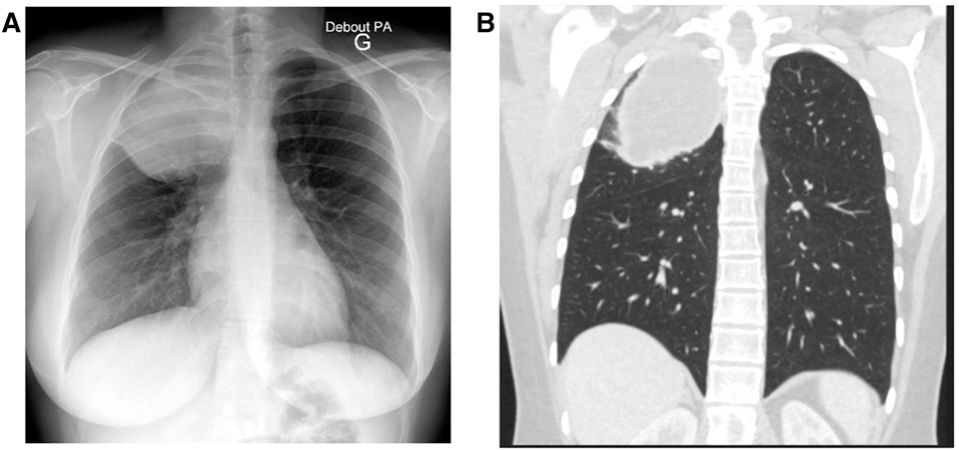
Chest radiography (Panel A) and computed tomography scan (Panel B) showing a cyst-like mass with smooth borders and no calcification in a Turkish woman presenting with a 1-month history of chest pain.
